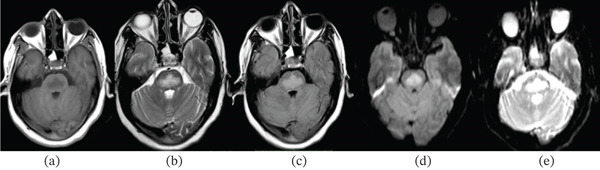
(a–e) T1, T2, FLAIR, DWI, and ADC images of the pontine lesion demonstrating hypointensity on T1, hyperintensity on T2 and FLAIR, and restricted diffusion on DWI images without associated drop on ADC map, demonstrating T2 shine‐through.
